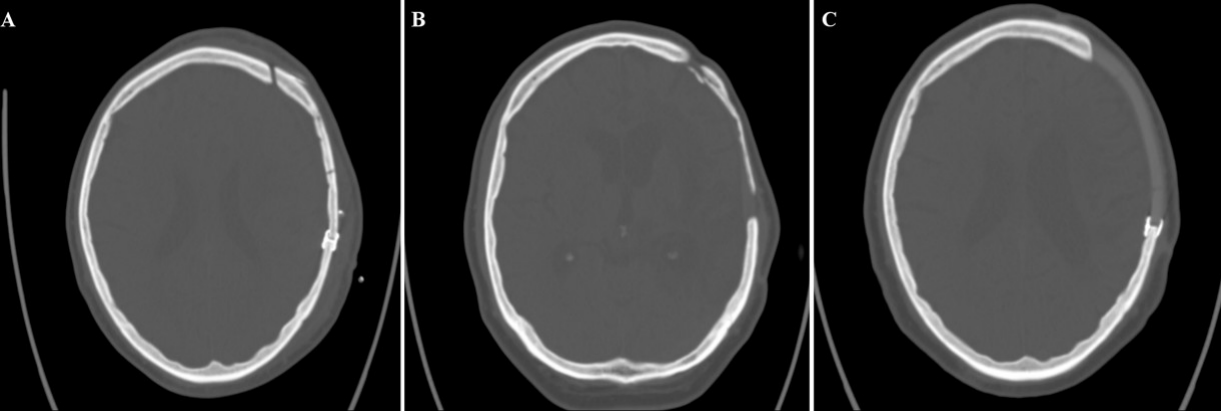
(A) Cranial computer tomography demonstrating the refixated bone flap directly after CP. (B) Same bone flap developing severe ABFR. (C) CT showing the subsequently replaced synthetic skull implant after reoperation.
